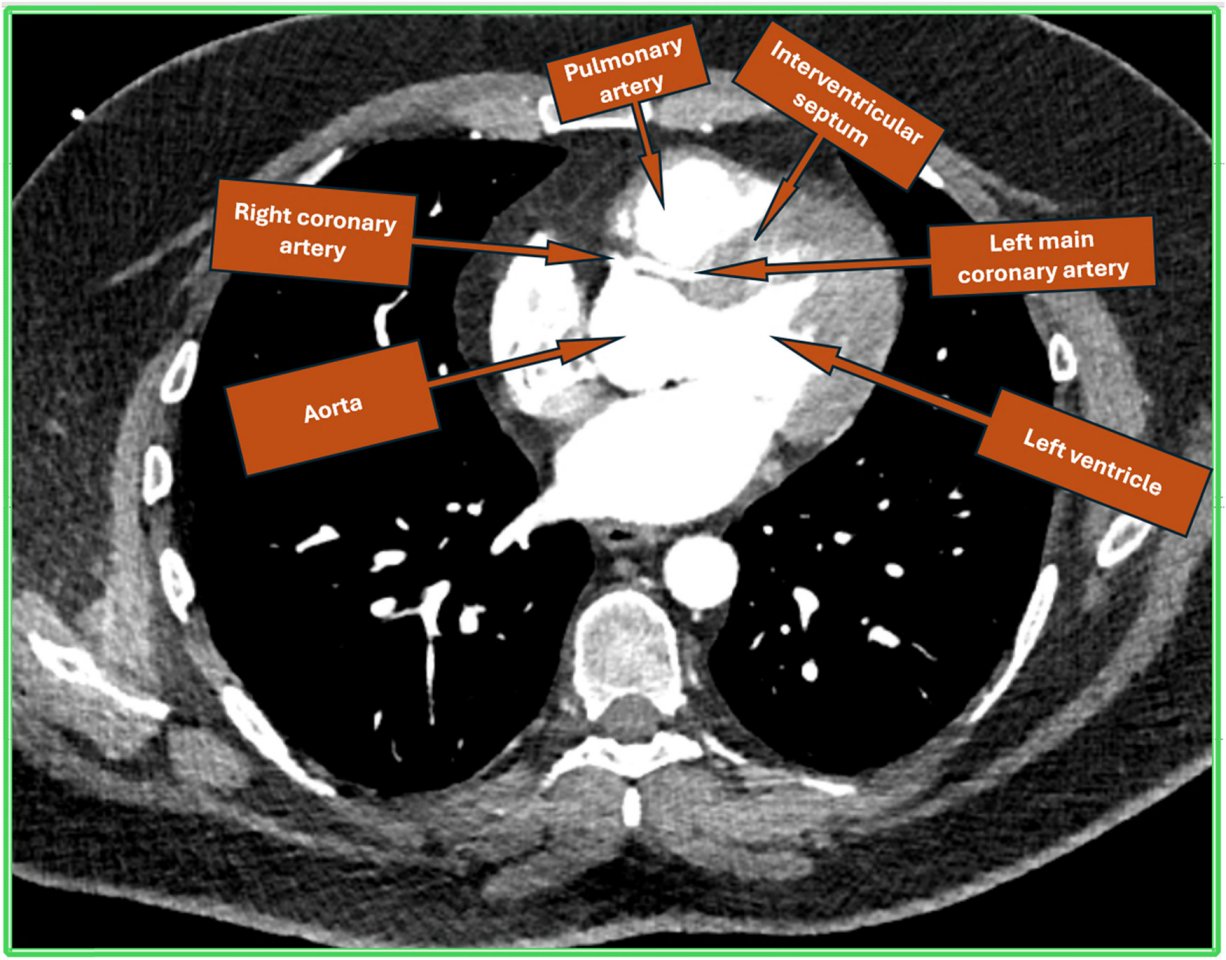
Cardiac computed tomogram showing single coronary origin from right coronary cusp and LCA having inter-arterial course between aorta and PA followed by course in the interventricular septum.
